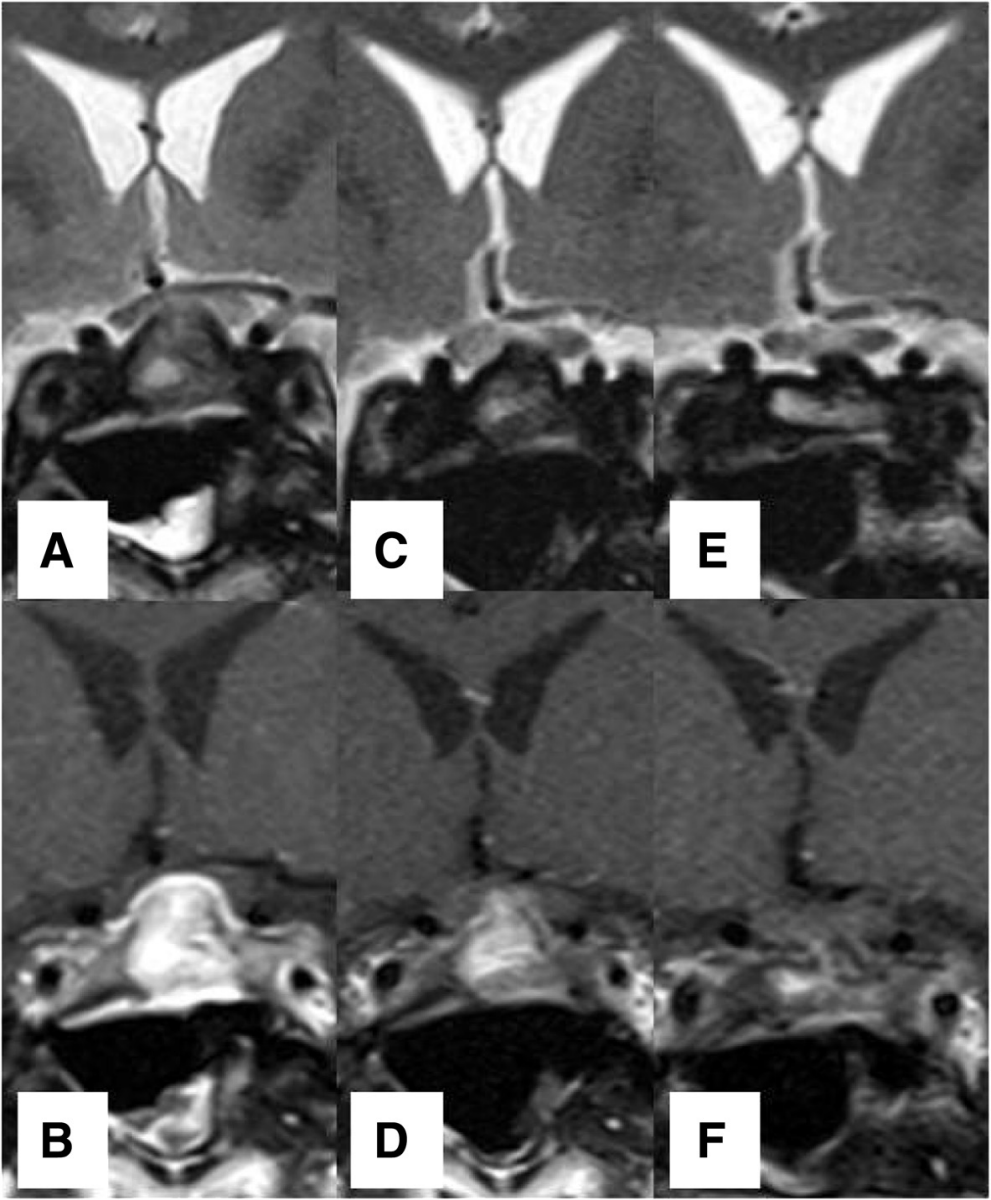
Magnetic resonance imaging of the pituitary. a-b T2W and T1W with Gadolinium contrast images on September 17, 2013. MRI scan showed sellar mass abutted to optic chiasm. c-d On September 2, 2014, the patient developed new temporal hemianopia. There was increase contrast enhancement in T1W and increase intensity in T2W at optic chiasm but slightly decreased in size of sellar mass. e-f On January 13, 2015, after treatment of high dose steroid, the sellar mass and intensity in T2W at optic chiasm were markedly decreased
